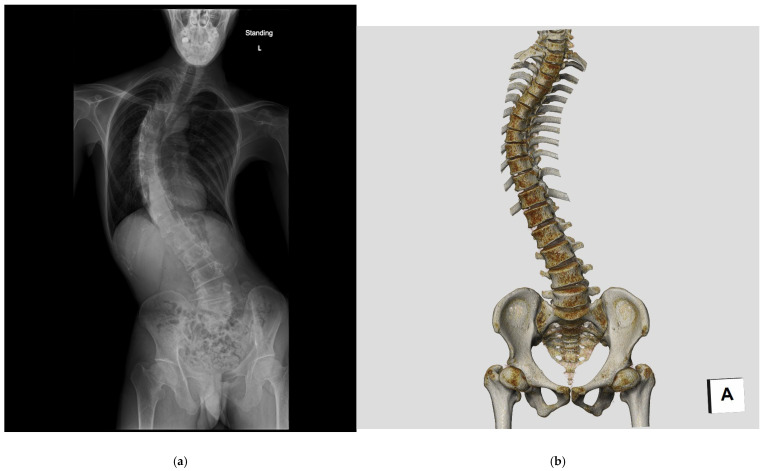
Radiographic and CT images of a patient with adolescent idiopathic scoliosis (AIS). (a) Standing postero-anterior (PA) radiograph of the whole spine. (b) Three-dimensional computed tomography (3DCT) image reconstructed using volume rendering.
